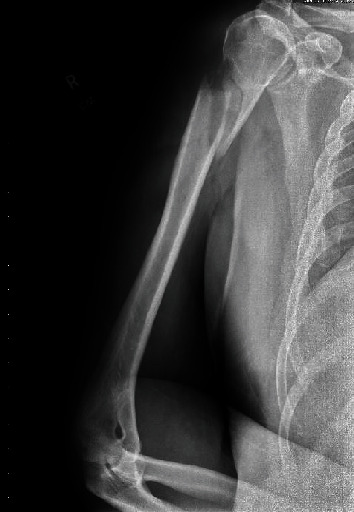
X-ray of the shoulder showing spiral fracture of proximal diaphysis of the humerus with displacement and angulation of the distal fractured bone.
